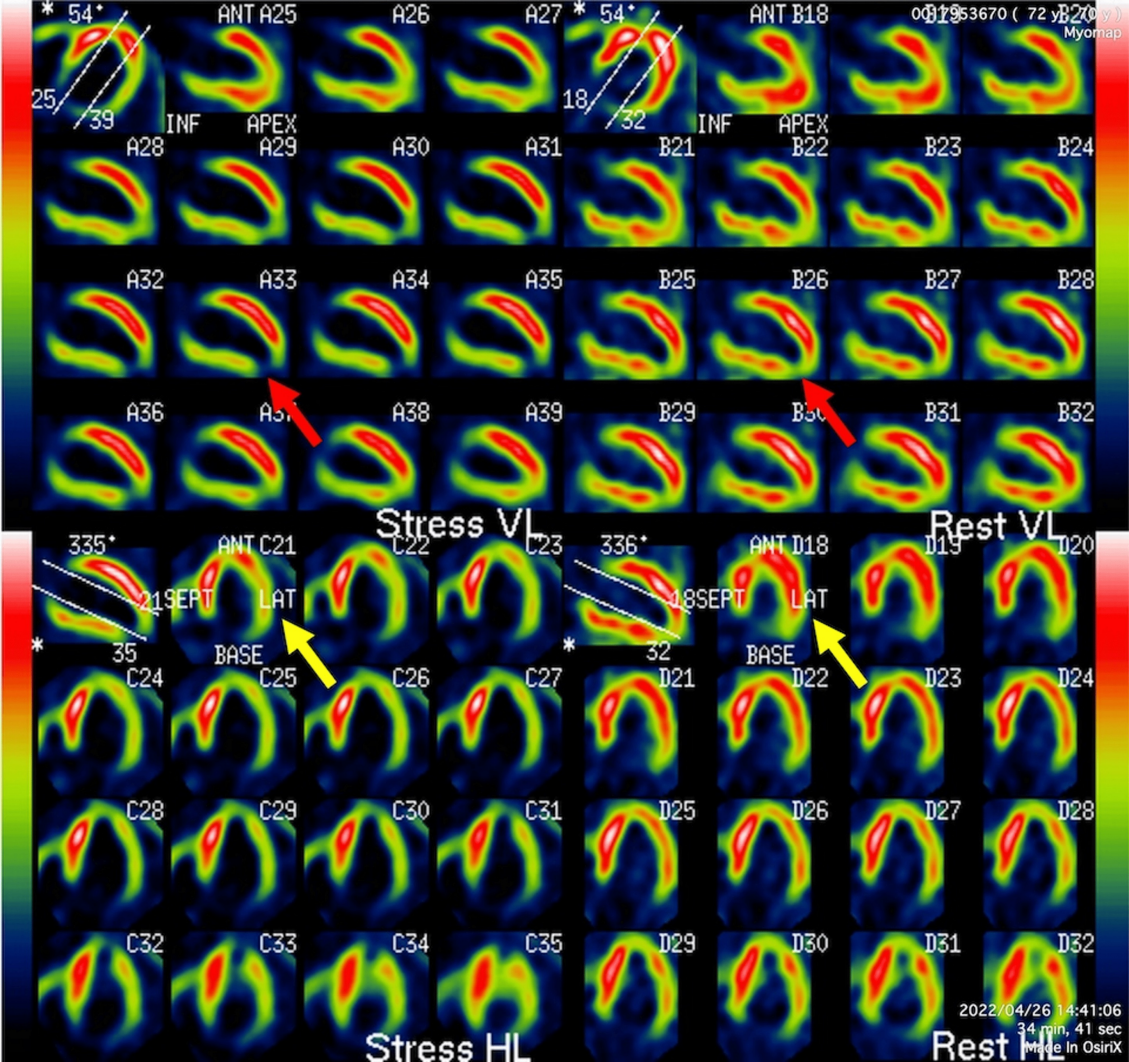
Myocardial single photon emission computed tomography Myocardial single photon emission computed tomography shows reduced blood perfusion at the apex both during exercise and at rest (red arrow). In addition, reduced blood perfusion is observed during exercise in the LAT (yellow arrow). Left ventricular myocardium other than the apex is shown to have viability because perfusion is maintained at rest (red and yellow arrows). The total perfusion deficit is 13%. VL: vertical long, HL: horizontal long, LAT: lateral wall
